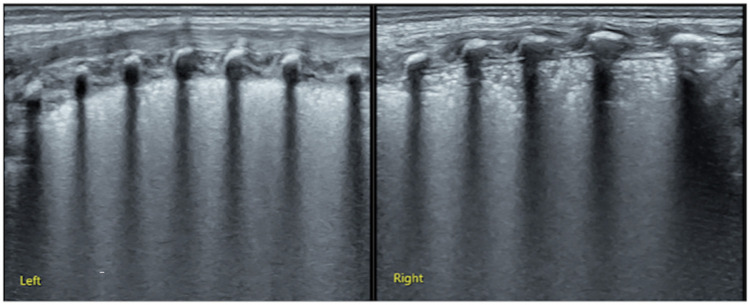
LUS examination results of Supplementary Video S1 (case 1). LUS showed snowflake-like lung consolidation in both lungs which is the typical ultrasound imaging of RDS, confirming that this patient needed PS treatment. LUS, lung ultrasound; RDS, respiratory distress syndrome; PS, pulmonary surfactant.
